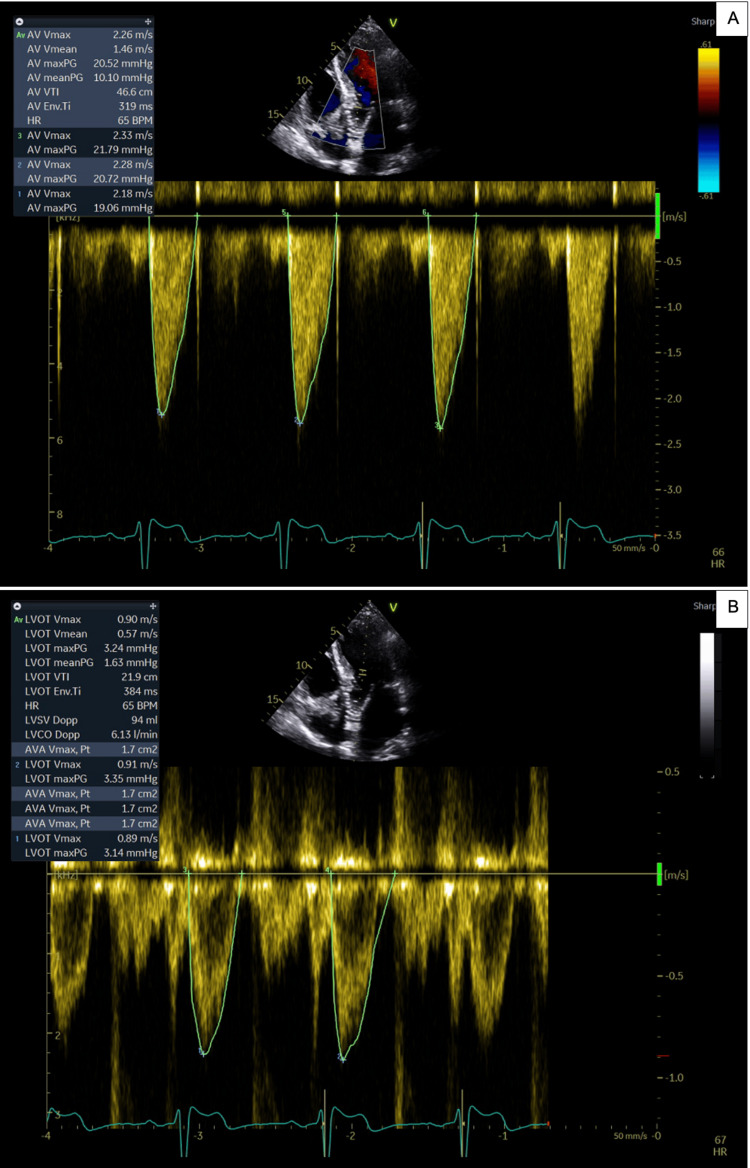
Post-TAVI echocardiogram demonstrating a well-seated valve (A) Continuous-wave Doppler showing normal aortic Vmax (normal <2.5 m/s). (B) Pulsed-wave Doppler demonstrating an aortic valve area of 1.7 cm² [6]. TAVI, transcatheter aortic valve implantation
